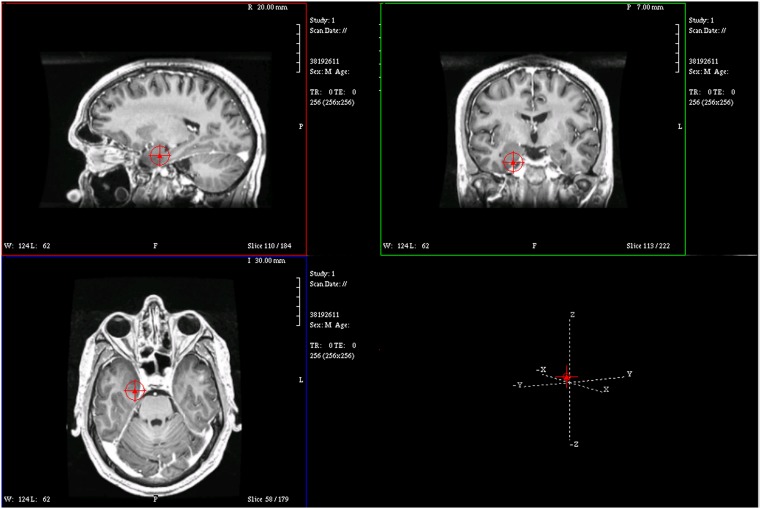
Magnetic resonance imaging plot of ECD of spike from Figure 1.
